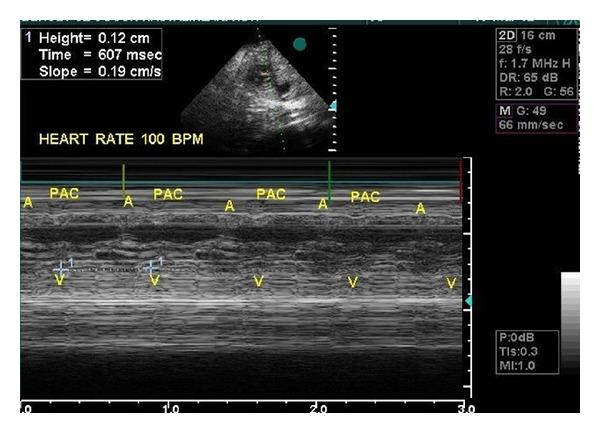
M-mode fetal echocardiogram showing bigeminal PACs associated with a fetal ventricular rate of 80–100 bpm (the A-PAC interval is markedly shortened).
